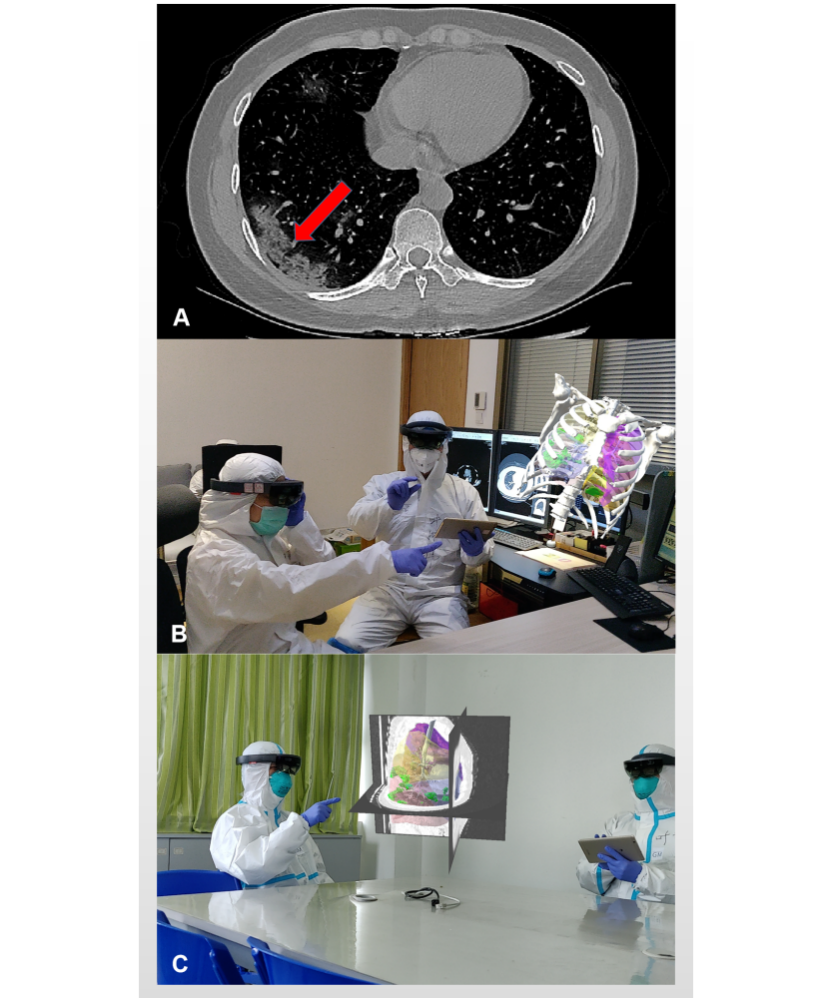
A 3D hologram of the pulmonary lesions caused by COVID-19. (A) A computed tomography scan of a patient with COVID-19 revealed patchy pure ground-glass opacities (red arrow). (B) A hologram can clearly show the lesion site and lesion range (green color) of the patient's lung infection, allowing stereoscopic viewing from 360 degrees in a physical world. (C) Simultaneous analysis of 2D and 3D images.
